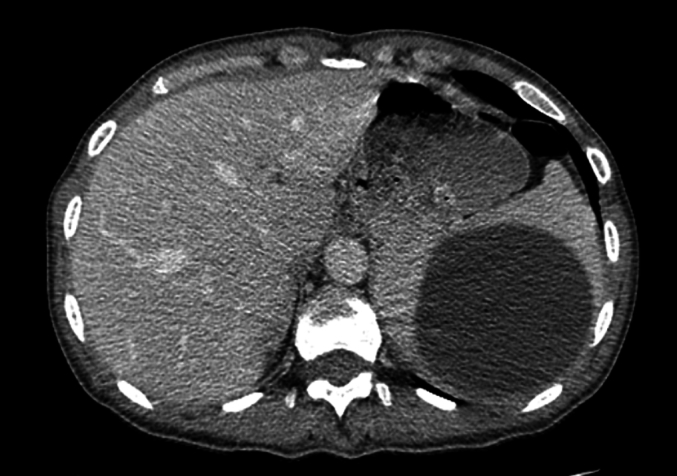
CT axial scan view appearance of a primary splenic hydatid cyst.
